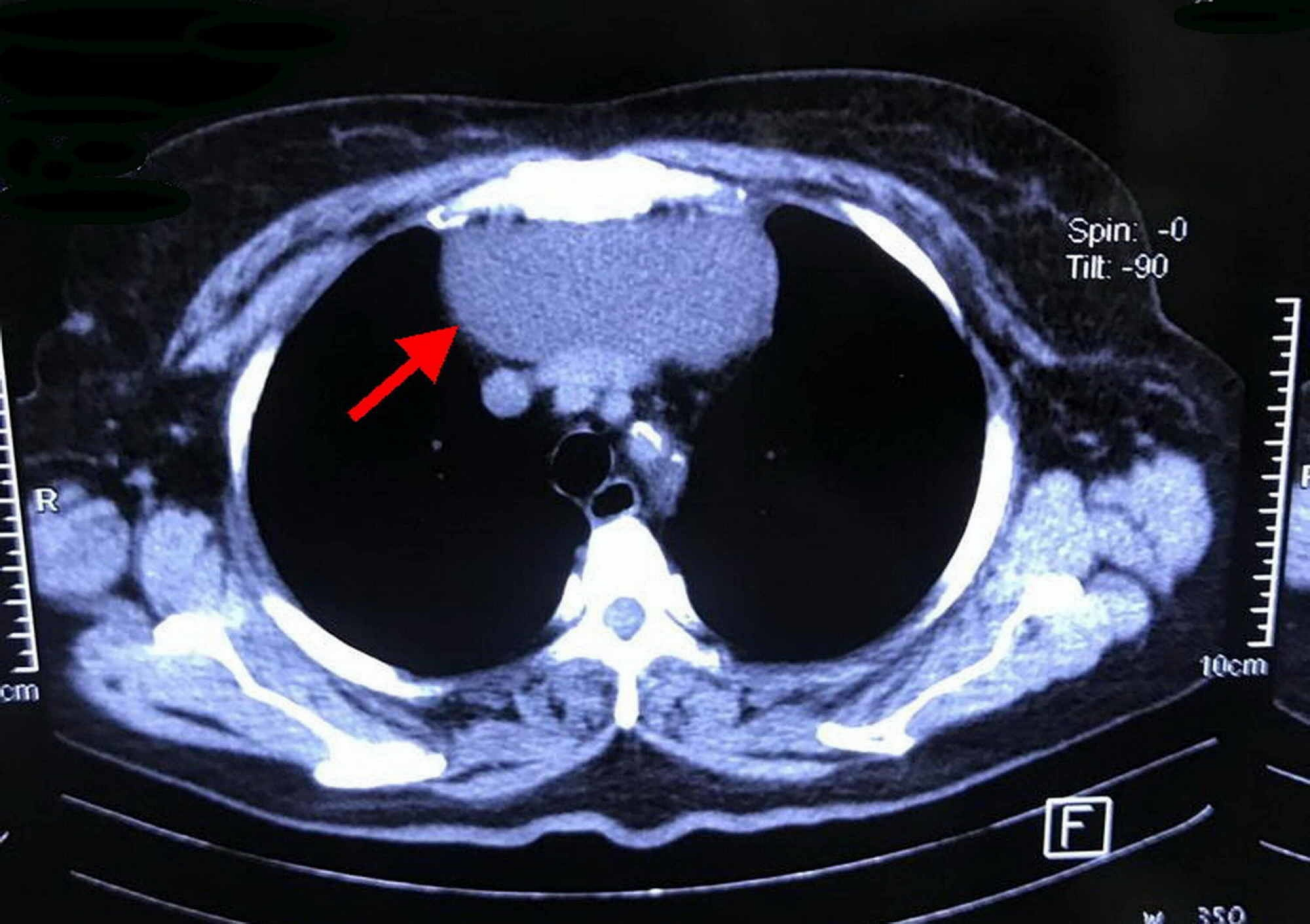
CT of the chest showing a cystic mass in mediastinum (red arrow).
